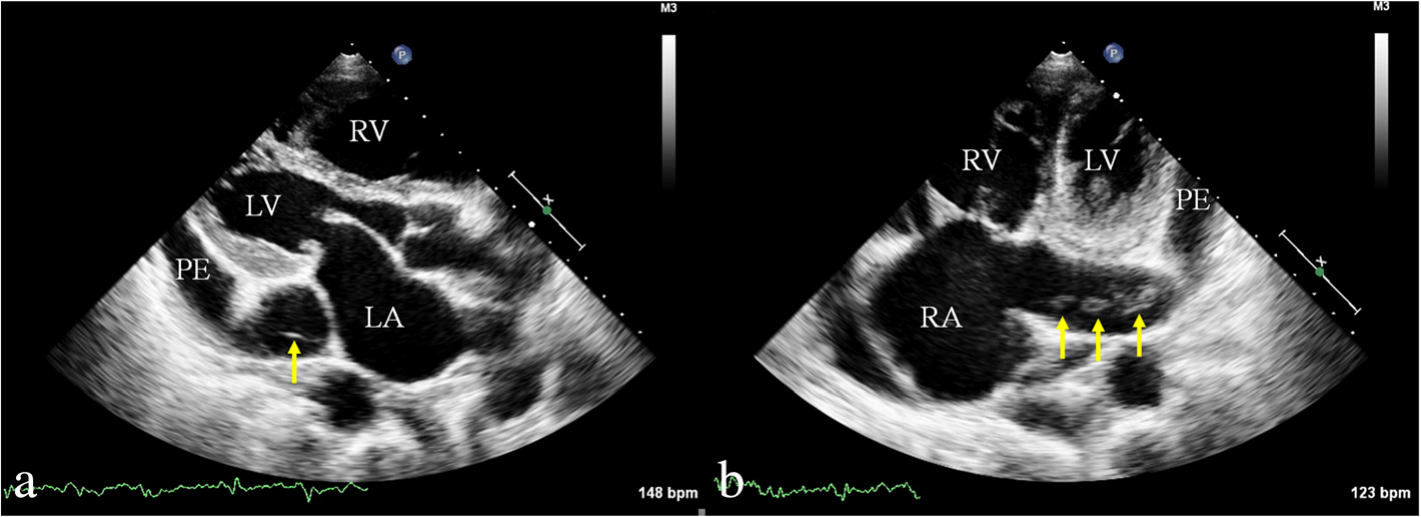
The aneurysmal dilated coronary sinus with the banded medium-echo mobile structure (yellow arrow). a in the parasternal left ventricle long-axis view. b In the modified apical 4-chamber view. CS: Coronary sinus; LA: Left atrium; LV: Left ventricle; PE: Pericardial effusion; RV: Right ventricle
